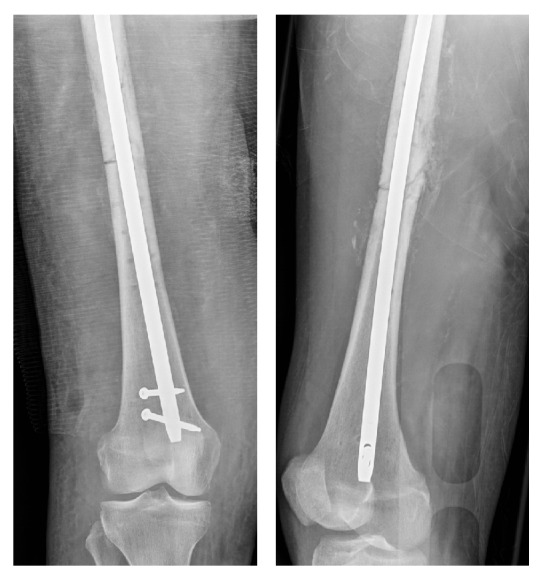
AP and lateral radiographs after revision fixation with an intramedullary nail.
